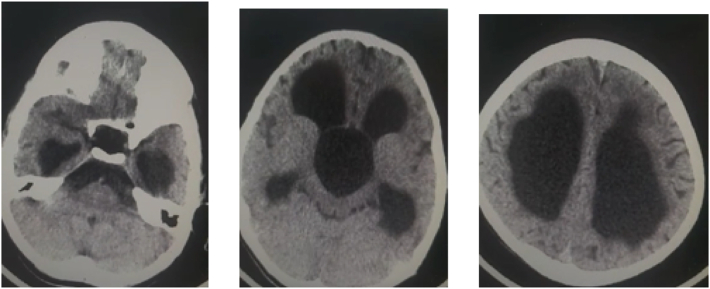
Non-contrast CT showing cystic lesion within 3rd ventricle causing severe hydrocephalus.
